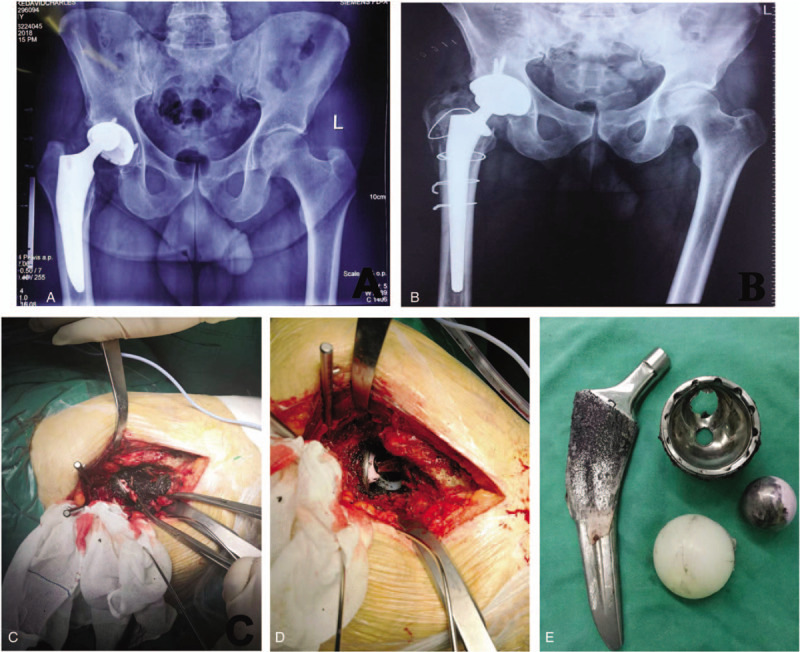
(A) Preoperative anteroposterior pelvis radiographs of the pelvis. (B) Postoperative anteroposterior pelvis radiograph following revision. (C) Copious amount of periprosthetic tissues presenting extensive metallosis. (D) The dissociated acetabular liner in surgery. (E) The destructed metallic shell and the protruded ceramic head through the defect.
